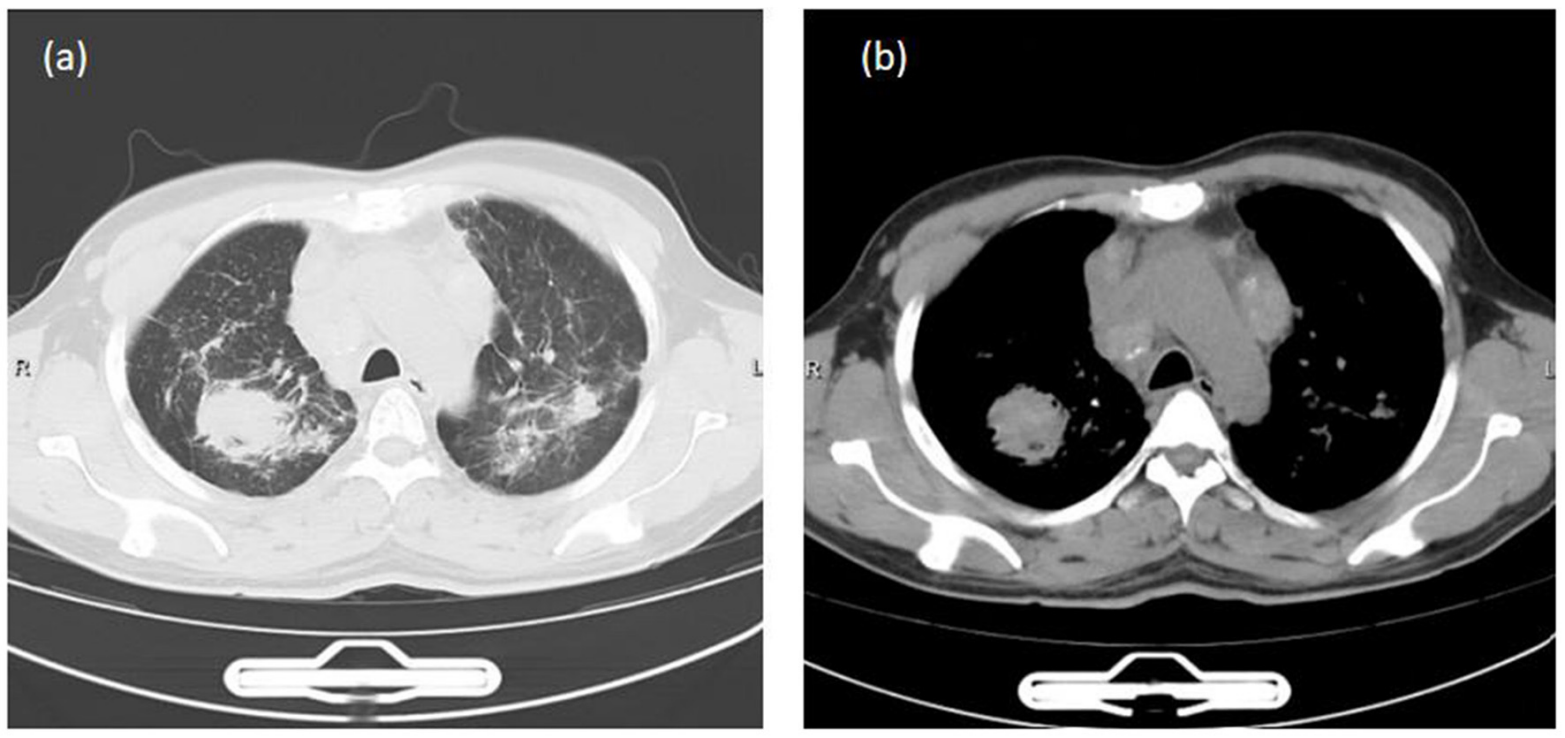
Chest computed tomography (CT) revealed multiple bilateral pulmonary lesions, multiple enlarged hilar and mediastinal lymph nodes, pericardial effusion, and lymphadenopathy in the cardiophrenic angle. (a) Lung window. (b) Mediastinal window.
